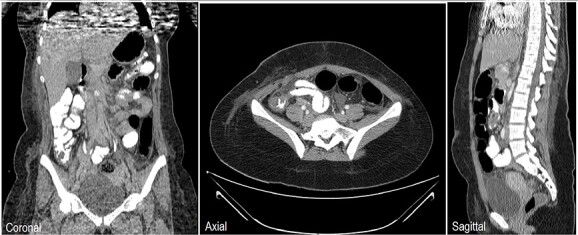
Chest, abdomen and pelvis CT scan with Oral & IV contrast postop Day 5 showed no contrast leak, no fistula and no obstruction at the site of surgery.
